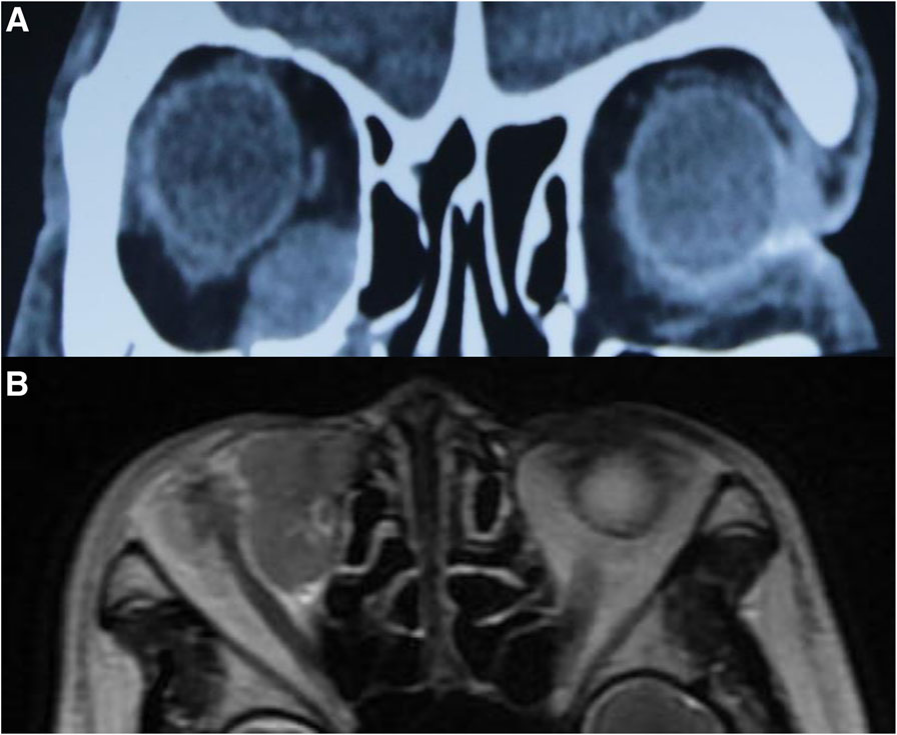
An orbit involved NUT midline carcinoma (NMC). a The orbital computed tomography (CT) and (b) Magnetic resonance imaging (MRI) showed an orbital mass extending to the adjacent paranasal sinuses
